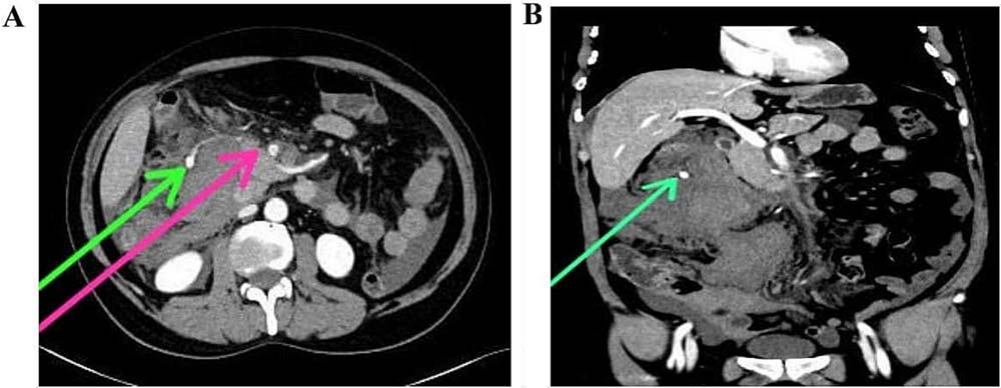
(A) Tiny branch originating from the SMA extending into the right side of the peritoneal cavity (pink arrow). A terminal outpouching/aneurysm (green arrow) shows contrast extravasation, suggesting the source of active arterial bleeding. (B) CT aortogram (coronal view, arterial phase) again demonstrates the aneurysm (originating from a side branch of the SMA) in the right hypochondrium, surrounded by hyperdense fluid/hemoperitoneum.
